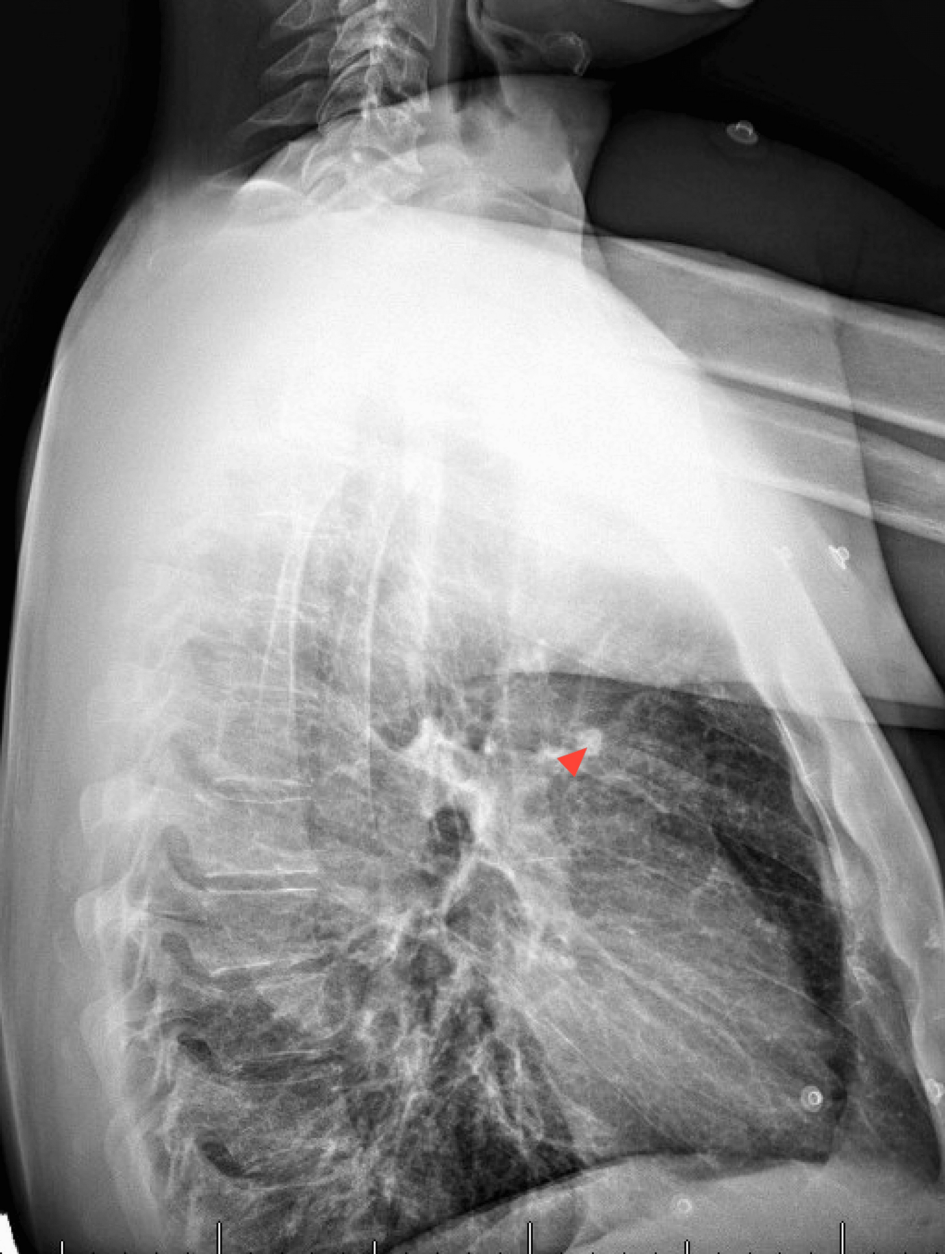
CXR lateral view. The red arrowhead shows a calcified granuloma in the left lobe. CXR, chest X-ray.
